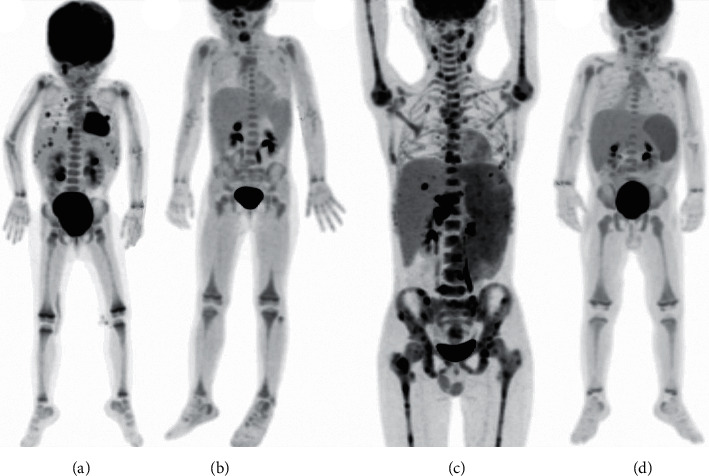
^18^F-FDG PET/CT maximum intensity of sHLH in 4 children. Non-Hodgkin's lymphoma (a), CAEBV (b), EBV-HLH (c, d), presenting as hepatosplenomegaly and increased FDG uptake of the spleen (a–d) and liver (a, c). (a) A 2-year-old girl with multiple enlarged lymph nodes (SUVmax:4.81) and multiple hypermetabolic lesions in extranodal organs, including brain, lungs, liver, and kidney (SUVmax:11.50). The child died three months later after admission. (b) A 3-year-old girl with multiple small lymph nodes (SUVmax:1.68, which was lower than the liver) slightly increased FDG uptake in the bone marrow (SUVmax:1.77). The children survived until the end of follow-up after hematopoietic stem cell transplantation. (c) A 14-year-old boy with focus and defusing increased FDG uptake in the bone marrow (SUVmax:11.13). He died of multiple organ failure. (d) A 2-year-old boy with slightly increased FDG uptake (SUVmax:2.1) in the bone marrow was slightly lower than the liver. He was still alive until the end of the follow-up.
